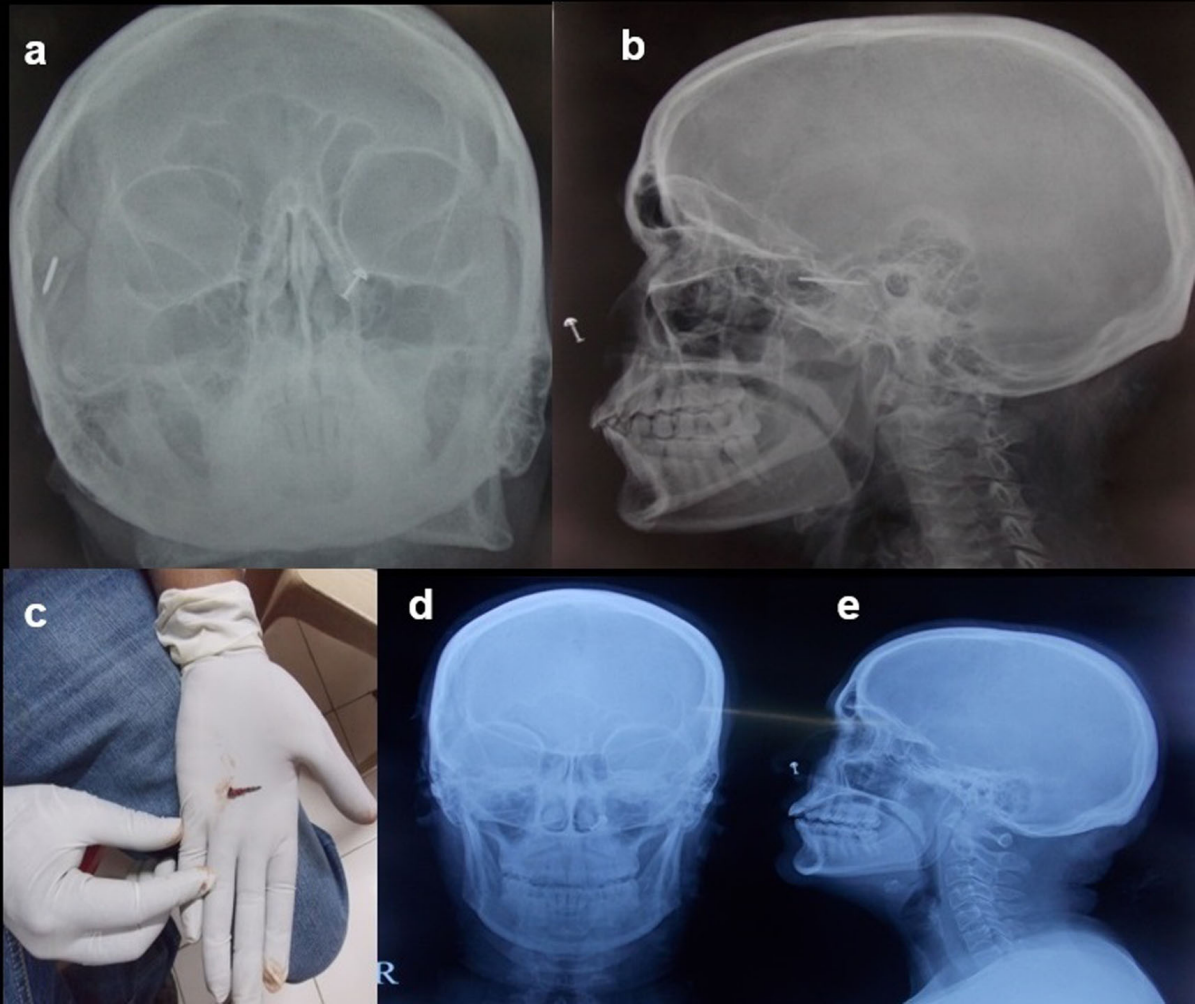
a-e: Radiograph skull A-P & Lateral view showed a radiodense nail-like structure on right side of scalp (a,b). The nail removed under ultrasound guidance (c). Normal radiograph skull A-P & Lateral view post removal of the foreign body (d,e).
